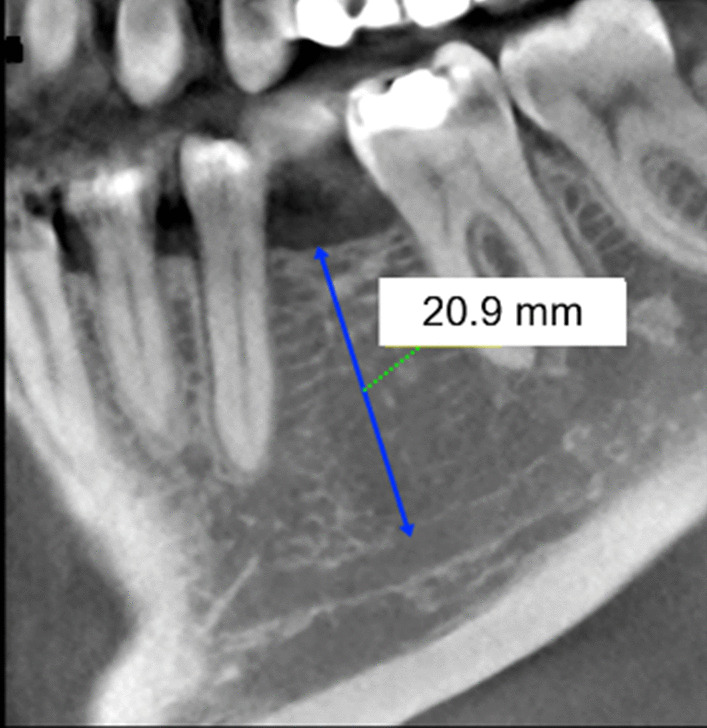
Sagittal plane from the CBCT of region 36 for case vignette 1. The alveolar ridge height is sufficient to place an implant
